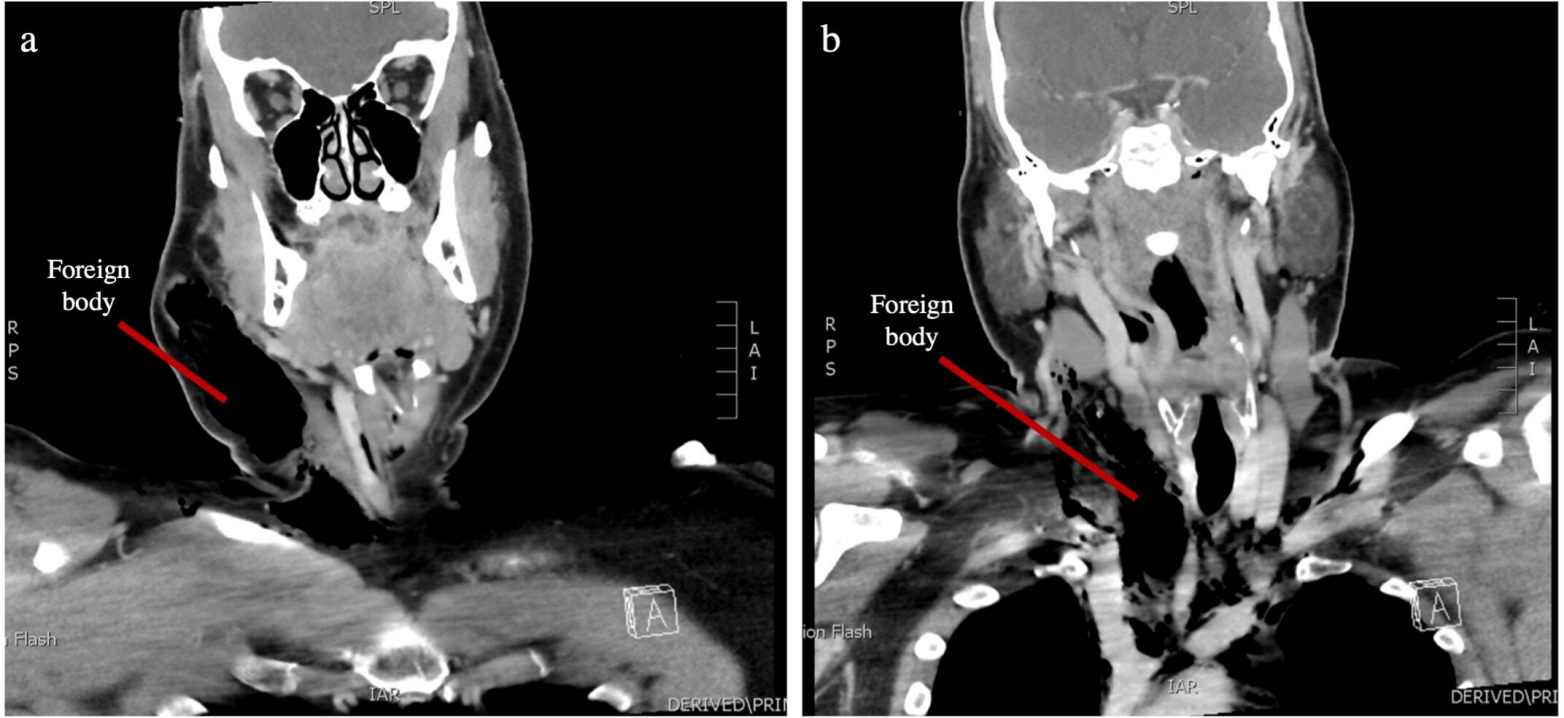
(a and b) Coronal CTA scans showing the hypodense wooden foreign body in the right neck.
